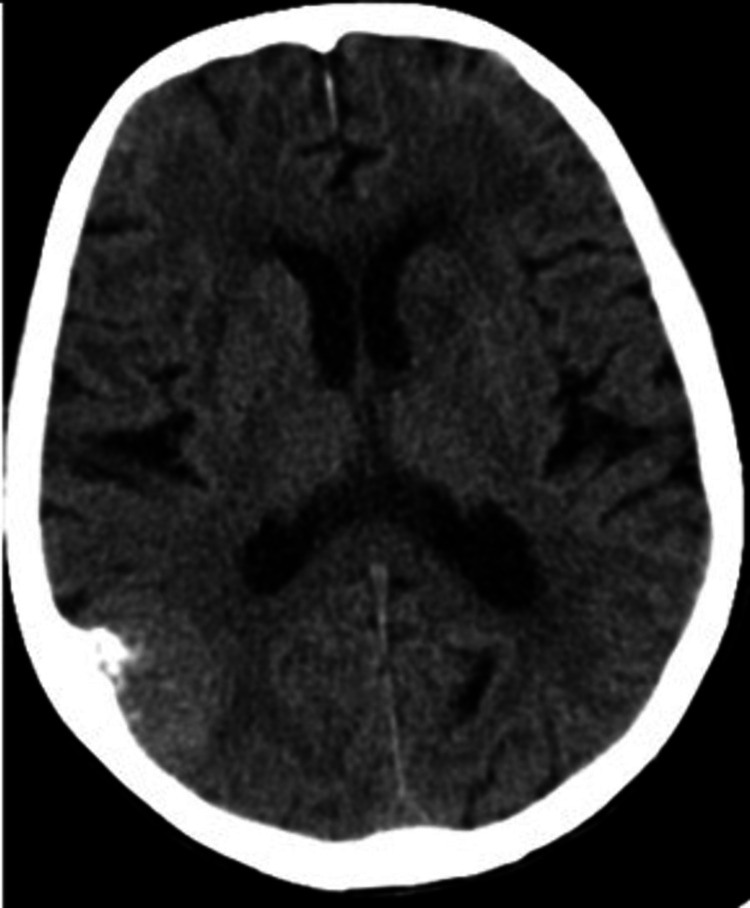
Cranial CT scan at admission, showing hypodense lesions in the anterior regions of both temporal lobes and a meningioma of the right parietal lobe. CT - computerized tomography
